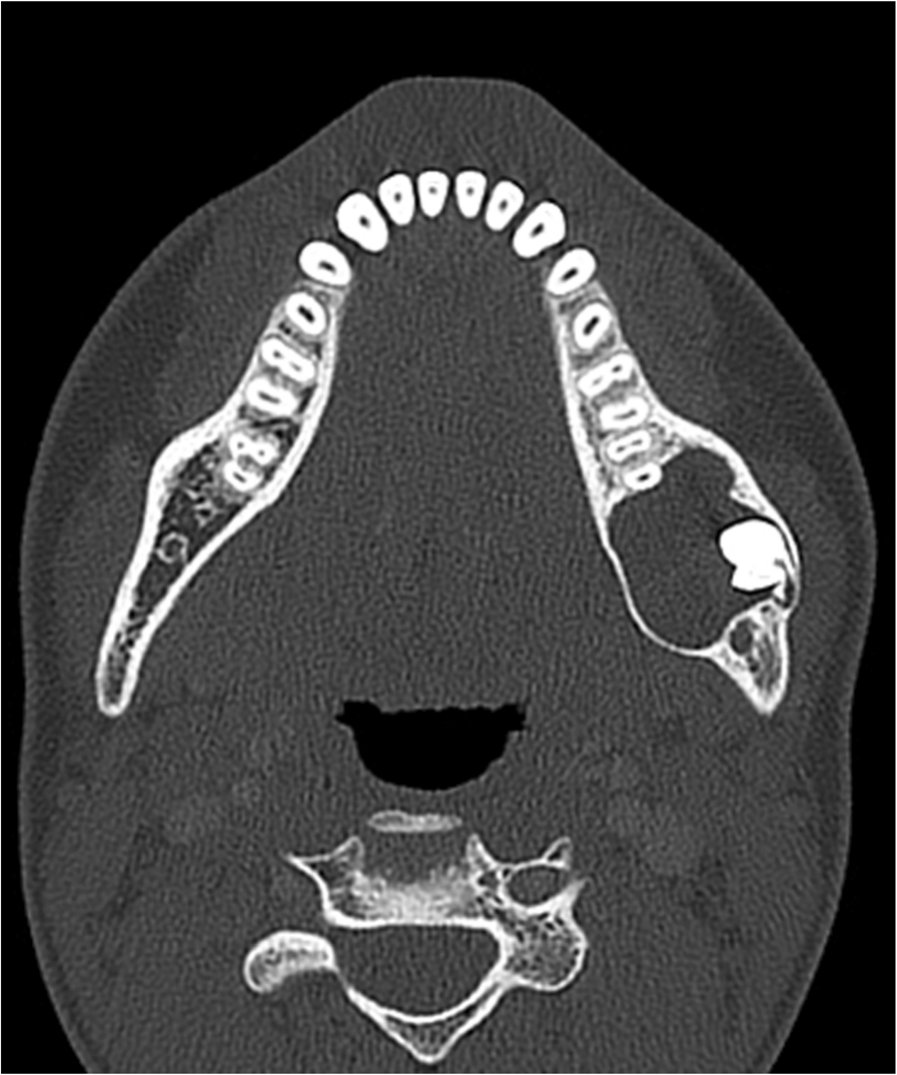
Odontogenic keratocyst. Axial CT image shows a well-defined expansile lucent lesion in the posterior left mandibular body surrounding the crown of an unerupted molar tooth
